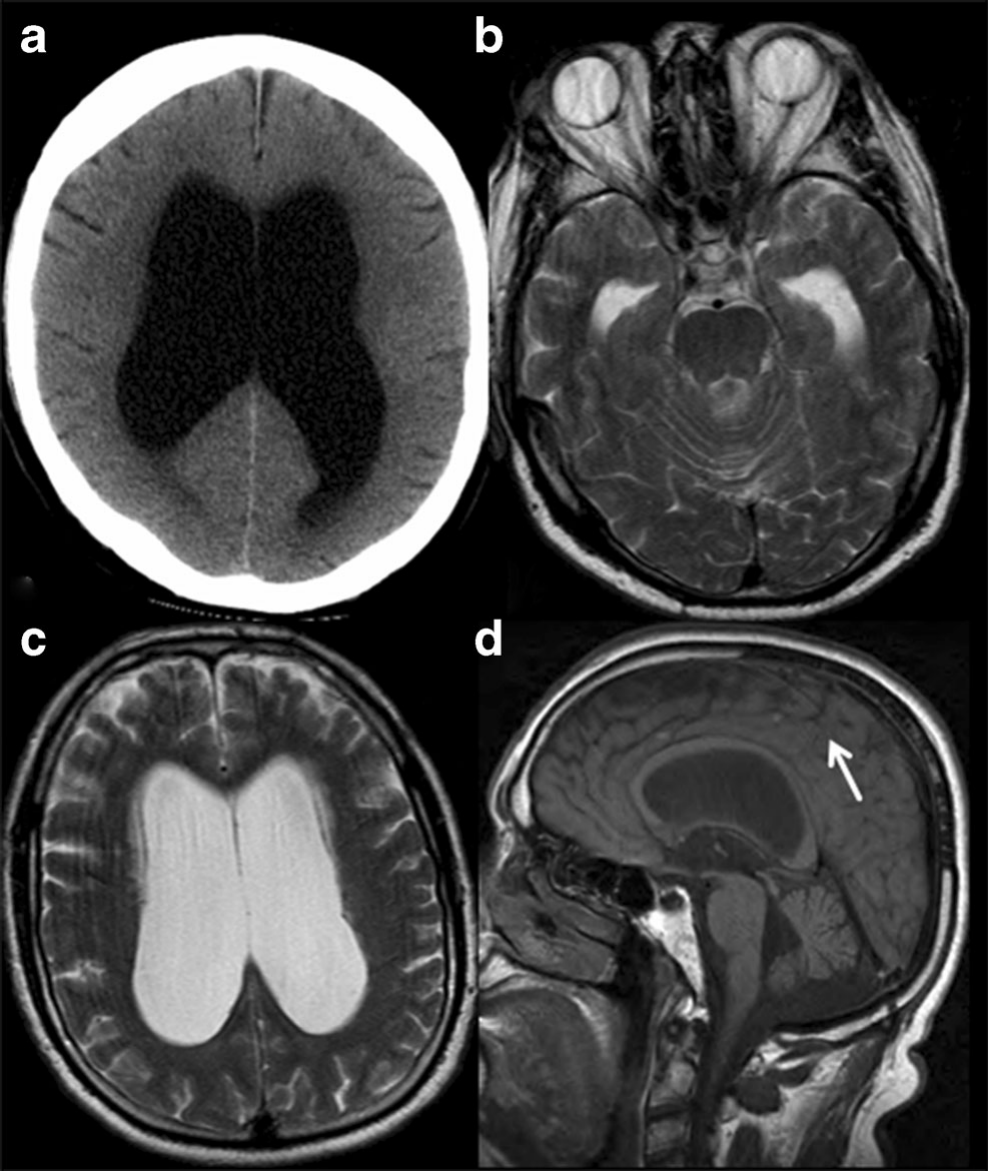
Normal Pressure Hydrocephalus (NPH). A 75 -ear-old male presented with progressive gait ataxia and memory loss. a axial CT section of the brain shows dilated lateral ventricles with effaced sulci. b & c axial T2W images of brain at the level of ventricles demonstrate dilatation of all the ventricles with prominent CSF flow void in the fourth ventricle (*black arrow*). d paramedian sagittal T1W section reveals ballooned corpus callosum with effacement of sulci towards vertex (*white arrow*)
